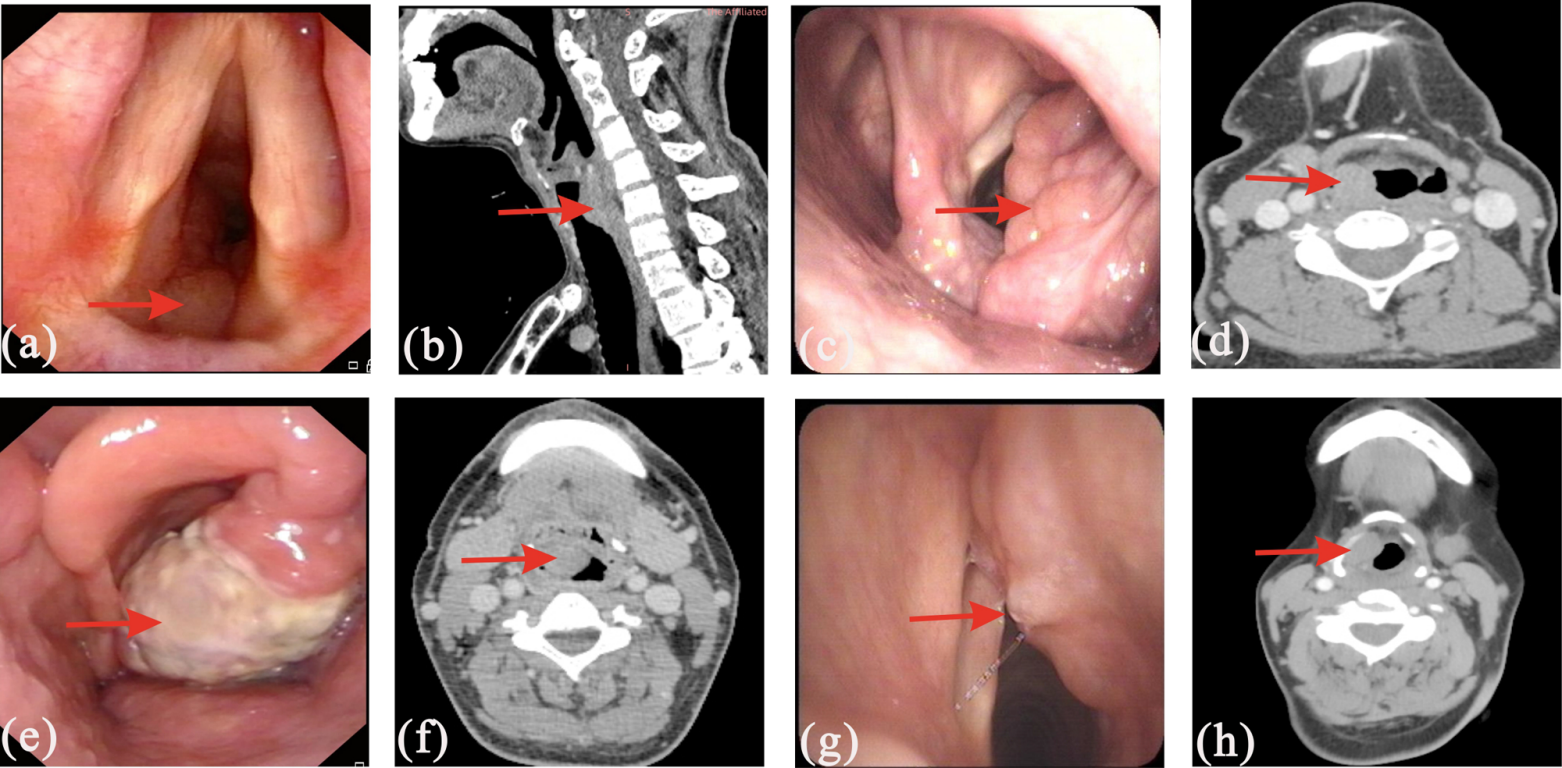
(a) Laryngoscopy indicated a non-smooth neoplasm in the subglottic region. (b) CT image indicating a mass at the glottis and subglottis and opening of the trachea. (c) Laryngoscopy indicated a mass in the right aryepiglottic fold. (d) CT image indicating a mass in the right supraglottic portion. (e) Laryngoscopy indicated a non-smooth neoplasm in the right aryepiglottic fold. (f) CT image indicating a mass in the right glottic portion. (g) Laryngoscope indicated a non-smooth neoplasm in the right ventricular bands. (h) CT image indicating a mass in the right supraglottic portion.
